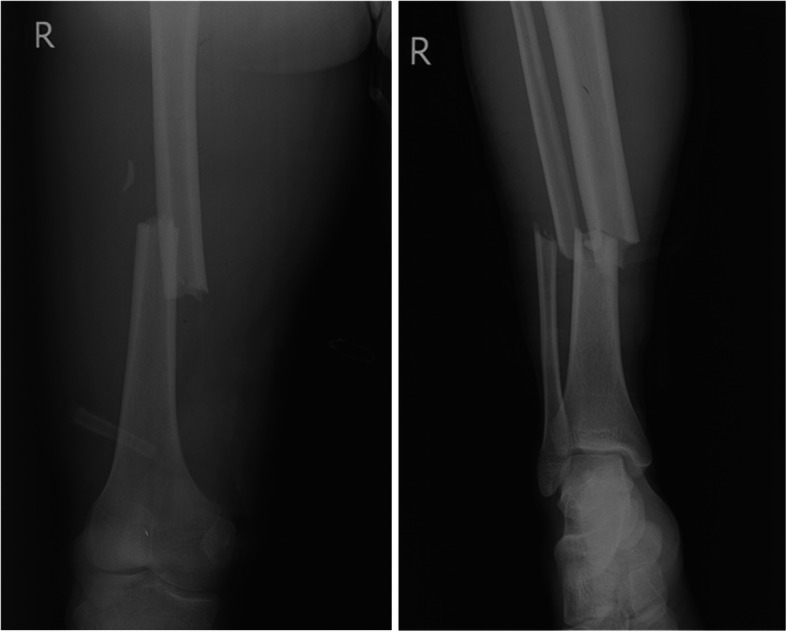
X-ray examination images of the right femoral shaft, as well as tibia and fibula obtained 2 h after the accident
